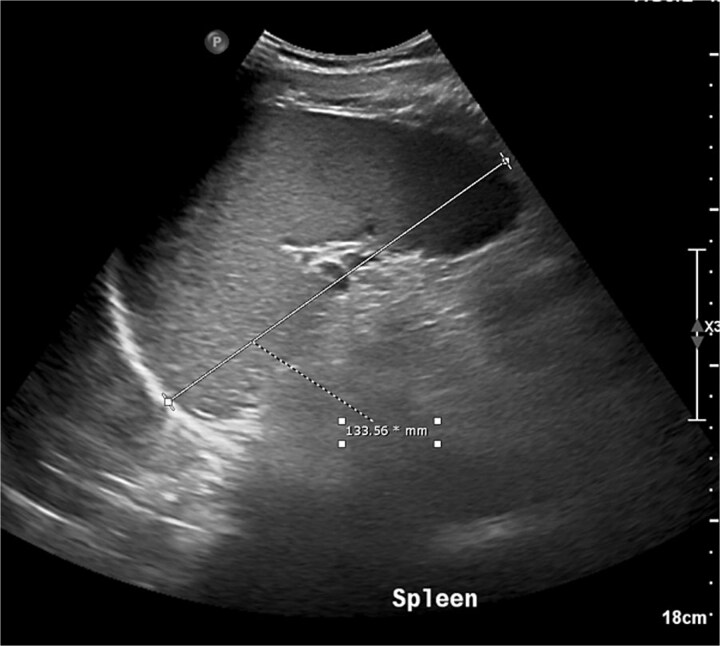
Grey scale ultrasound examination of the abdomen showed a mildly enlarged spleen.
